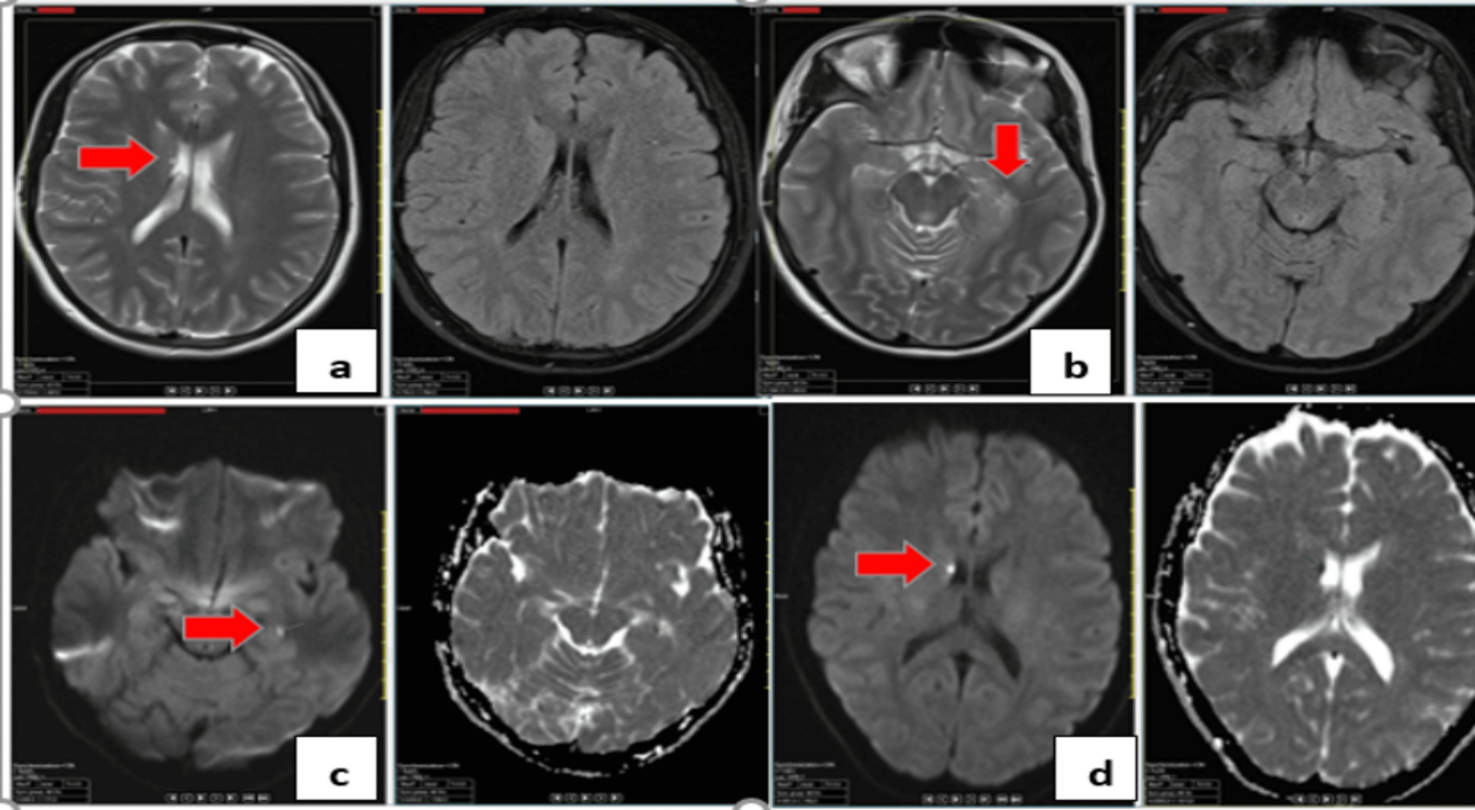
Cranial MRI a) T2A/FLAIR signal increase on the left ependymal surface and periventricular ependymal surfaces; b) edema in the occipital region; c) diffusion restriction in the left hippocampus and d) right caudate nucleus, and medial temporal lobe FLAIR: fluid-attenuated inversion recovery; MRI: magnetic resonance imaging
